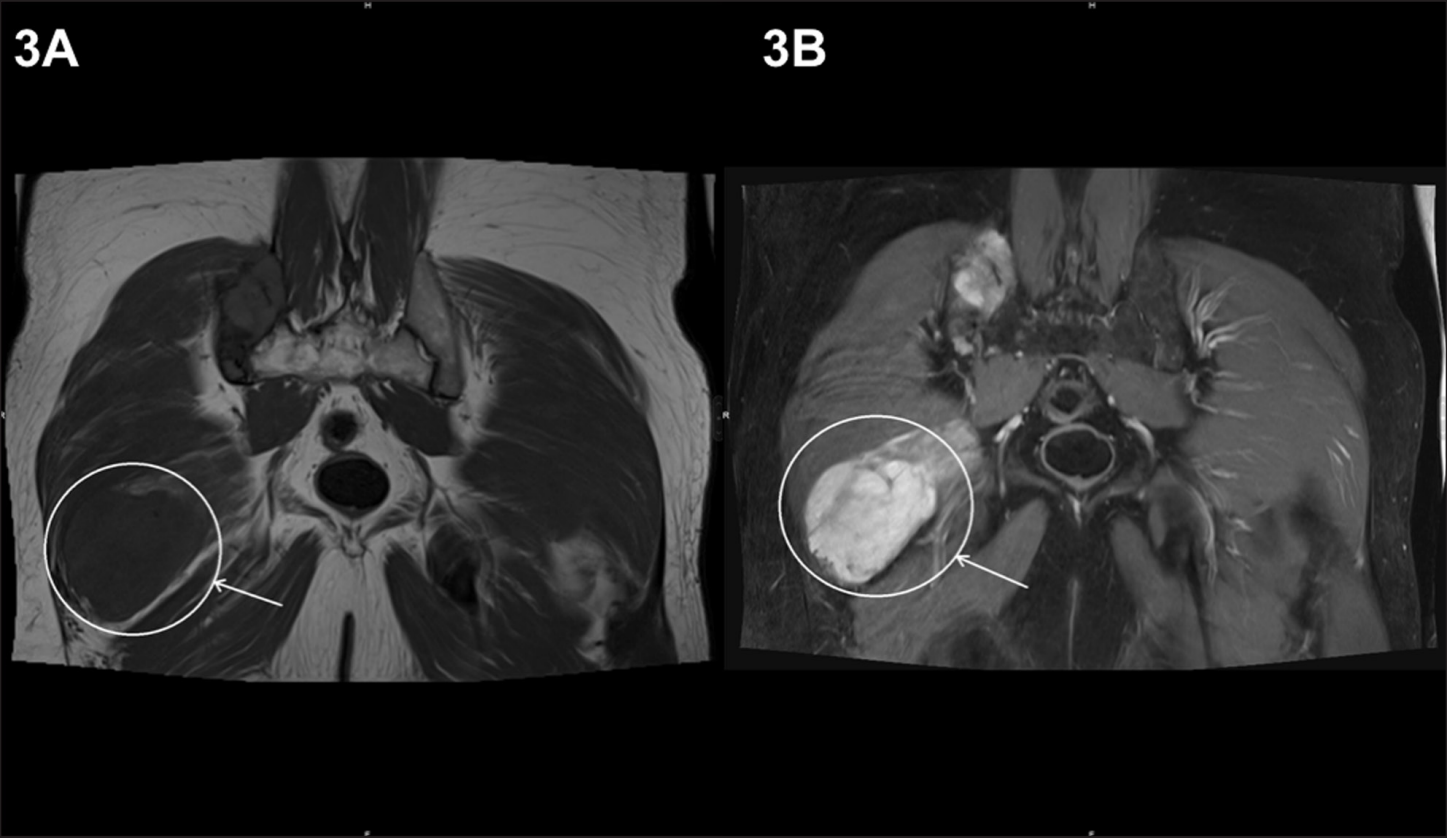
The diagnosis of fibrous dysplasia and intramuscular myxoma was retained also known as Mazabraud’s syndrome. Routine follow-up was started with a six-month MRI.
